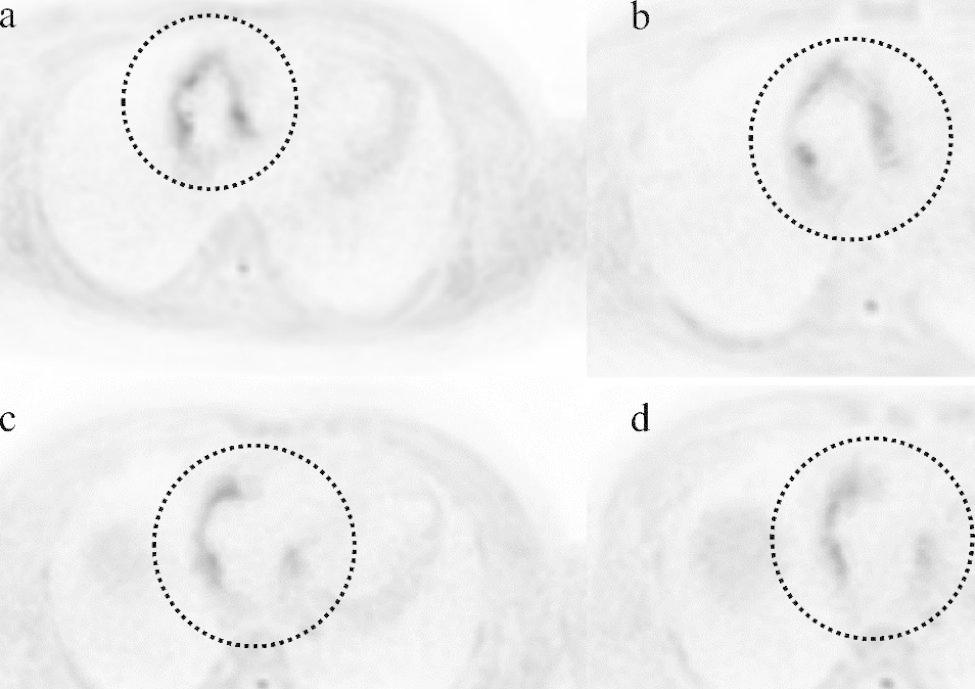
Diffusion-weighted whole-body magnetic resonance imaging with background body signal suppression The images are horizontal sections from a, b, c, and d arranged from cranial to caudal order. We could detect high-signal area, which CT was low density CT: computed tomography
